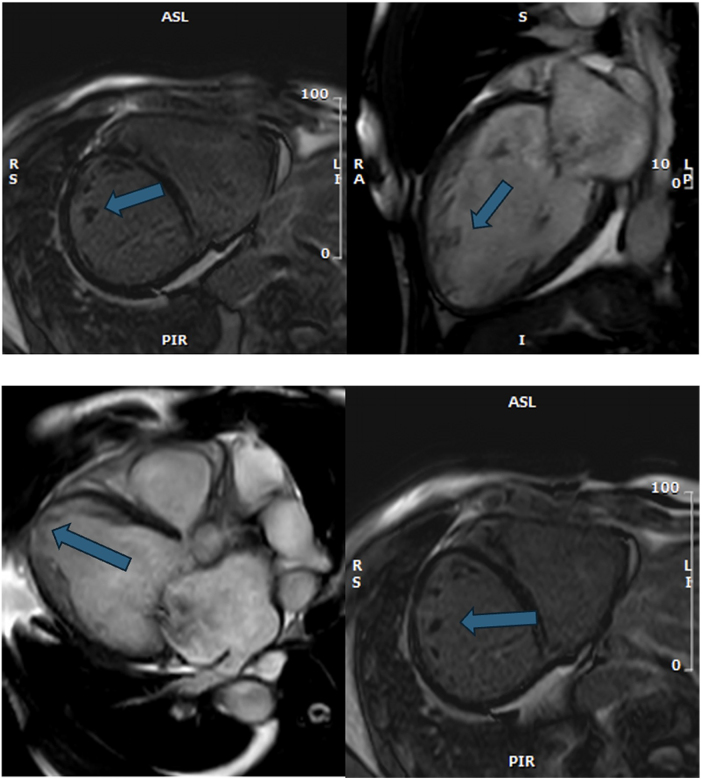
Cardiac MRI. Images (A, D) Hypertrabeculation in anatomical LV (short axis). Images (B, C) Hypertrabeculations are in the mid-apical part (sagittal localizer image).
